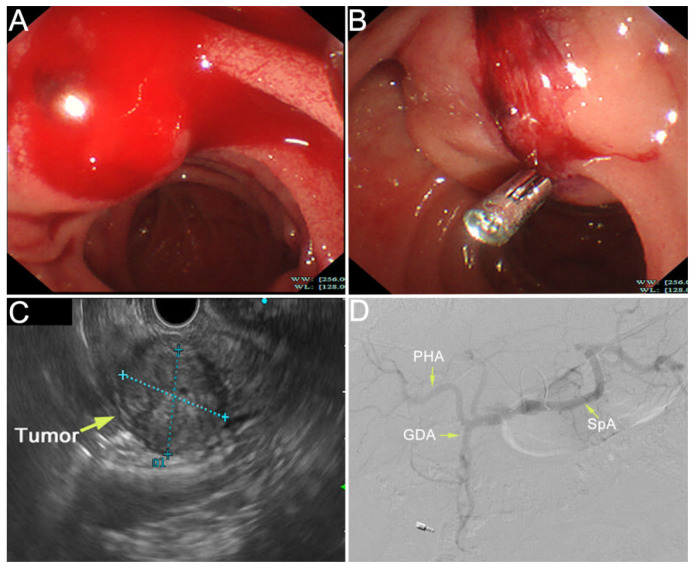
The duodenal papilla was plump with pulsatile bleeding by emergency duodenoscopy (A). The ampullary mass was observed by endoscopic ultrasonography, with a size of 22.7 × 28.2 mm (C). We diagnosed that the tumor had invaded the artery and the bleeding site was indistinct due to the persistent bleeding. Hemostasis was performed by using an endoscopic metal clip and the hemorrhage was partially relieved (B). However, due to the fragility of the tumor, complete hemostasis was difficult. Then, we tried to perform TAE in hemostasia. However, there were no obvious signs of contrast extravasation (D). After a Multi-Disciplinary Treatment (MDT), we decided to perform emergency pancreaticoduodenectomy, which was recommended by most experts on MDT. Intraoperatively, it was found that the tumor was located in the ampulla of vater and had invaded the pancreatic tissue. Modified Child anastomosis was performed after radical pancreaticoduodenectomy: gastrojejunostomy (Braun’s anastomosis), pancreaticojejunostomy (anastomosis of pancreatic duct with jejunal mucosa through a tent duct), and biliojejunostomy (end-to-side). He also underwent fenestration for a cyst in his right liver. The operation went well.
